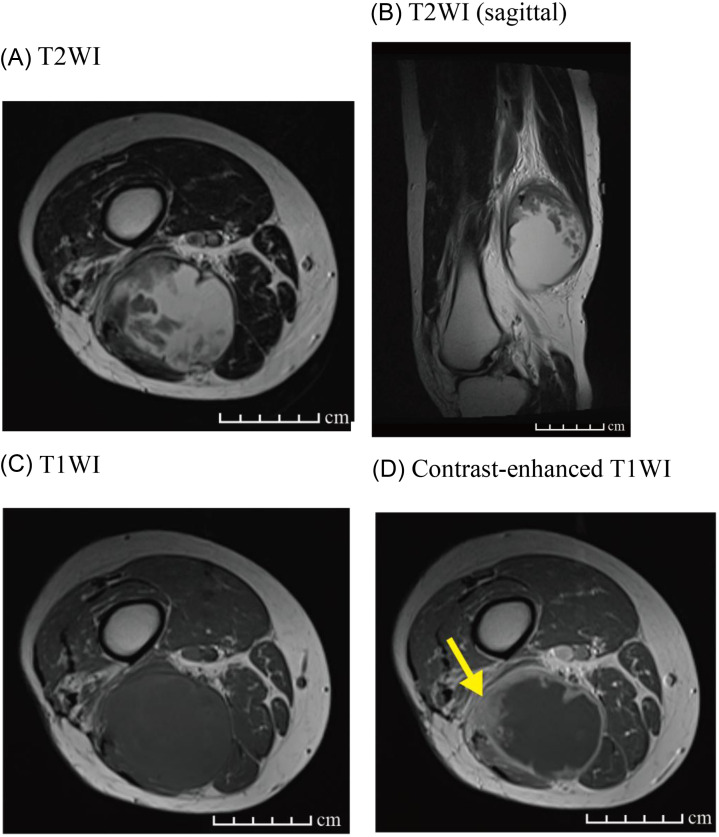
Case 3, a male in his 50s. Magnetic resonance imaging (MRI) is shown; (A) T2-weighted image (T2WI), (B) T2WI on sagittal plane, (C) T1-weighted image (T1WI), and (D) contrast-enhanced T1-weighted image (an enhancing mural nodule is indicated by the yellow arrow).
